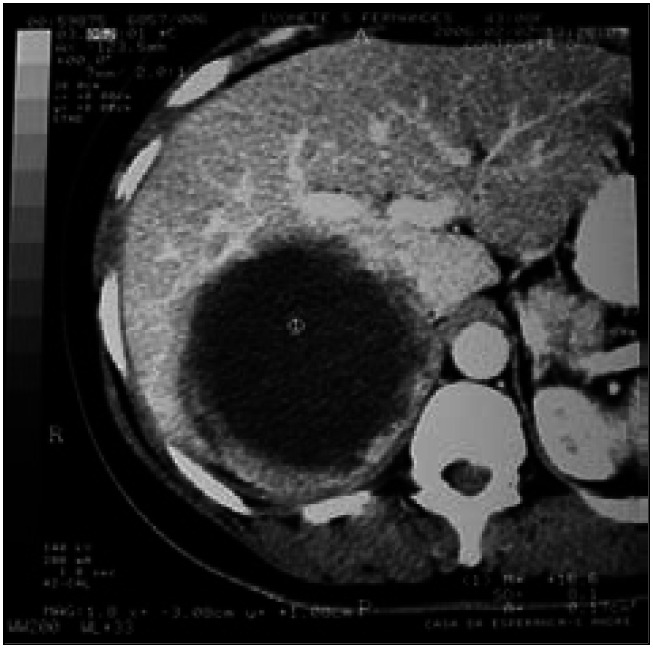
(Case 2) – Predominantly cystic retroperitoneal tumor that involved right hepatic lobe (computed tomography scan).
